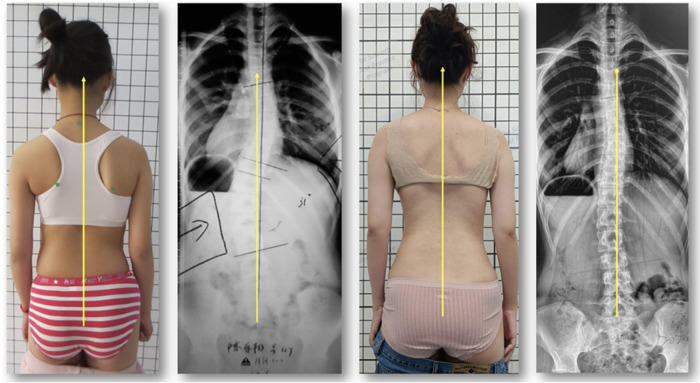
Girl with AIS 4C, born in 2004, in December 2016, the thoracic curvature was 25 degrees, and the lumbar curvature was 31 degrees at the start of treatment with a CAD/CAM chêneau derivative. The x-ray taken one year after full brace weaning (2020) showed a thoracic curvature of 11 degrees and lumbar curvature of 10 degrees. A symmetric trunk shape has been achieved as well.
